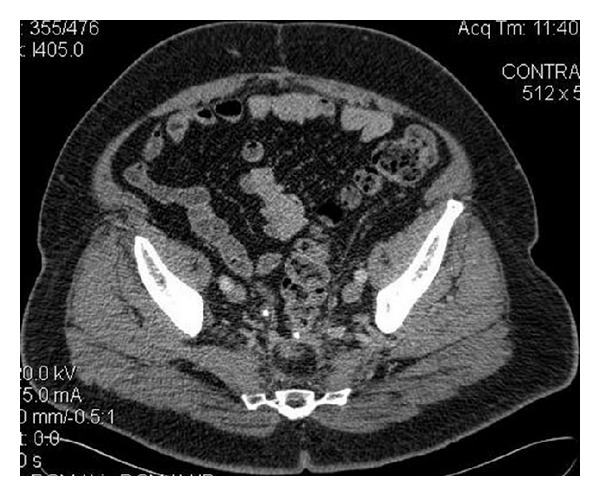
Computed tomography of the pelvis showing no evidence of extraureteral relapse.
